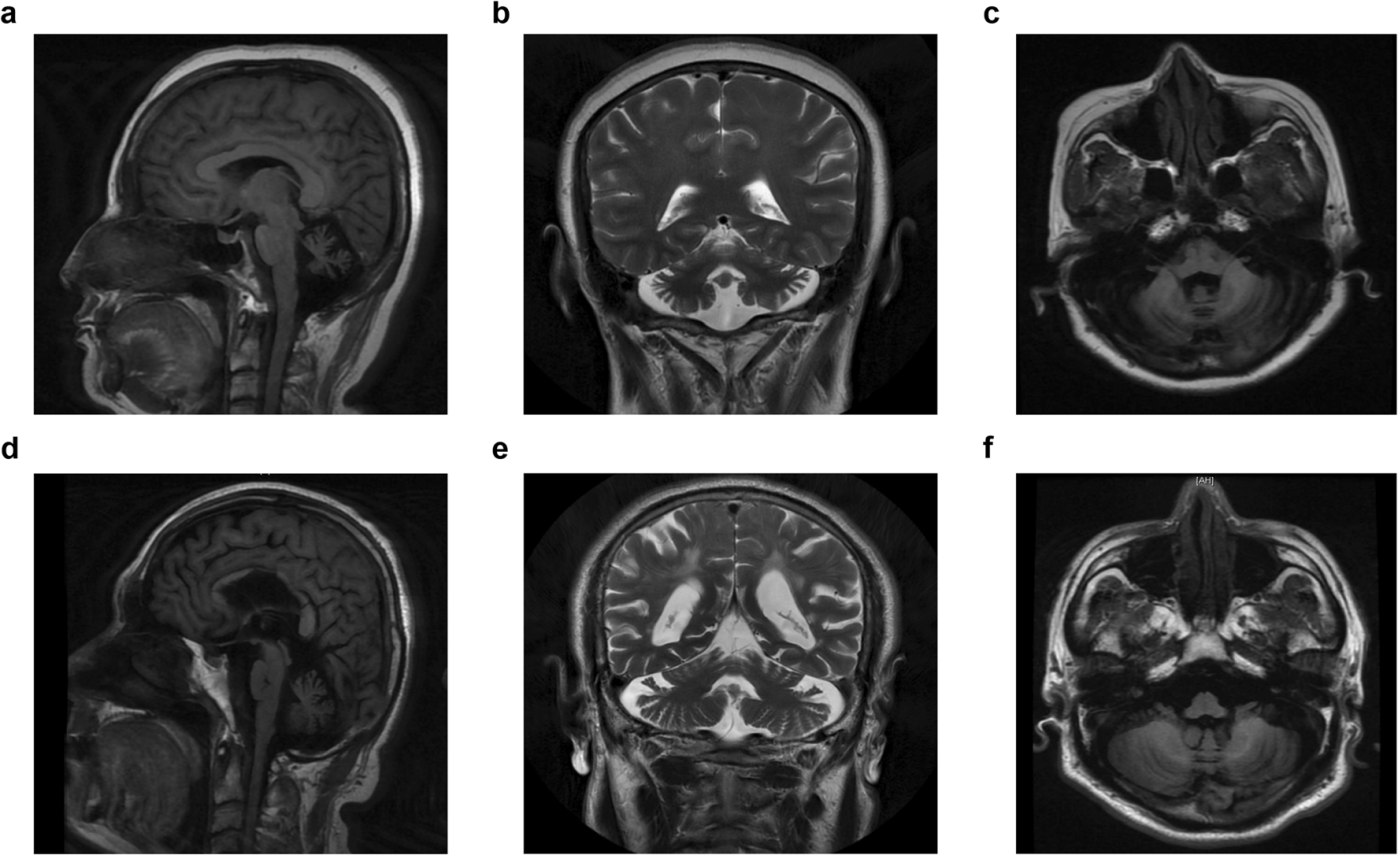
Brain MRI of patients with SCA5. Sagittal (a T1-weighted), coronal (b T2-weighted), and axial (c T1-weighted) brain MRI of the proband of the family with SCA5. Sagittal (d T1-weighted), coronal (e T2-weighted), and axial (f T1-weighted) brain MRI of the sporadic patient with SCA5. They both displayed moderate cerebellar atrophy
